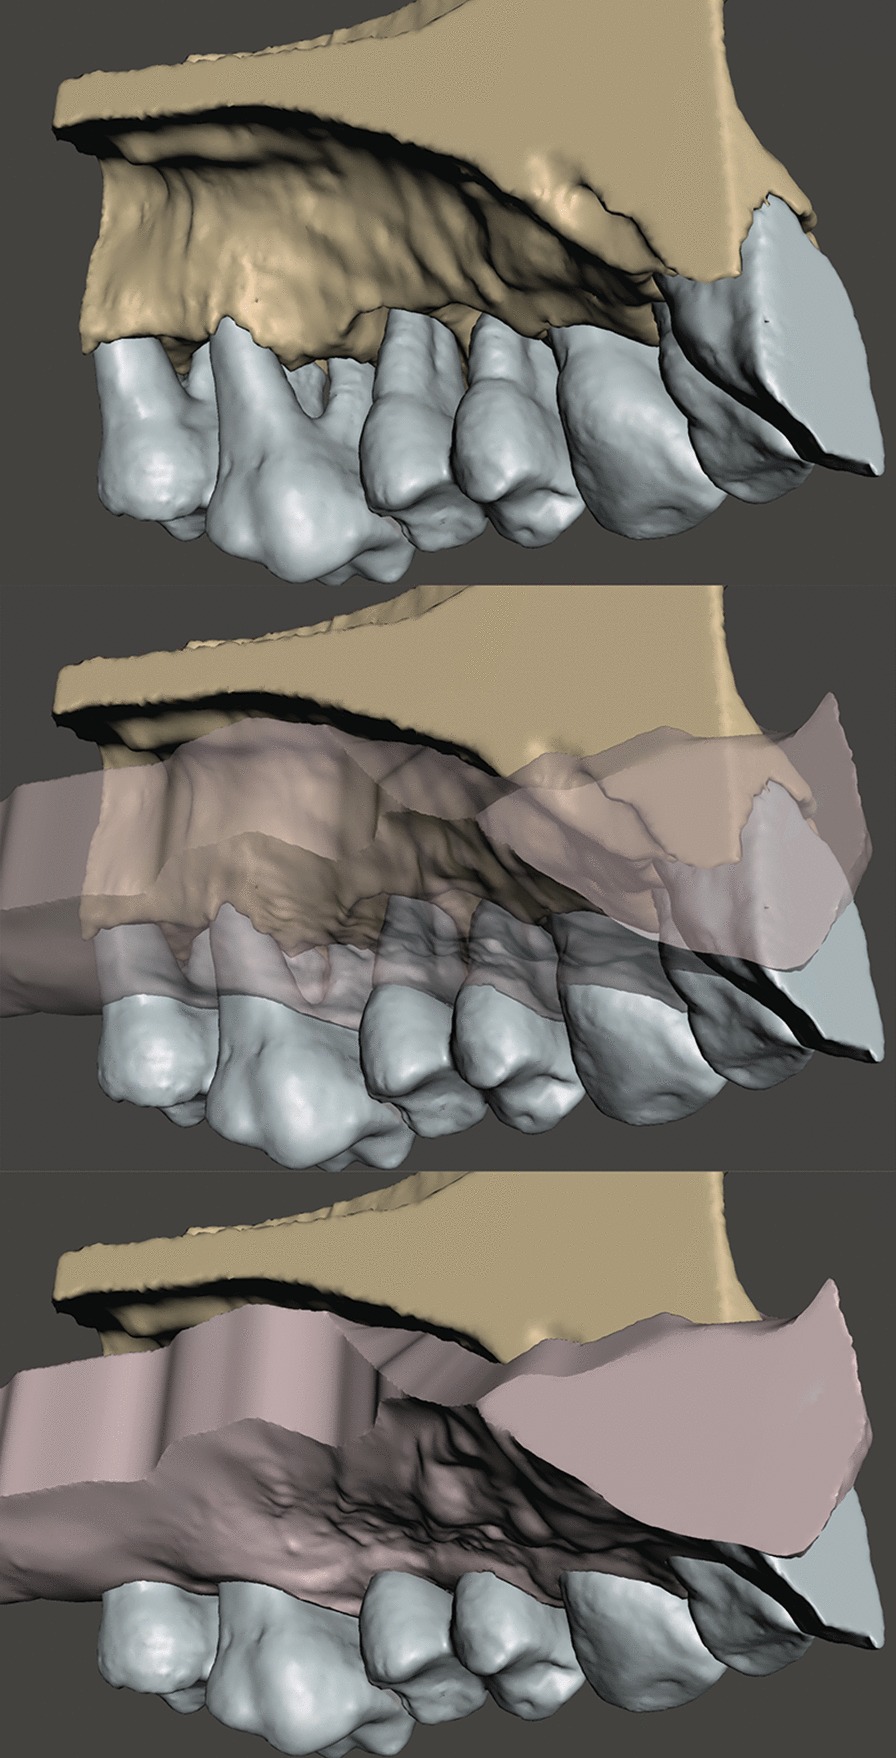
Soft tissue model derived from intraoral, hard tissue model reconstructed from CBCT. Circumdental crater around tooth 24 and 25, Class III furcation involvement of tooth 26, soft tissue model derived from an intraoral scan superimposed over the segmented model derived from CBCT dataset
